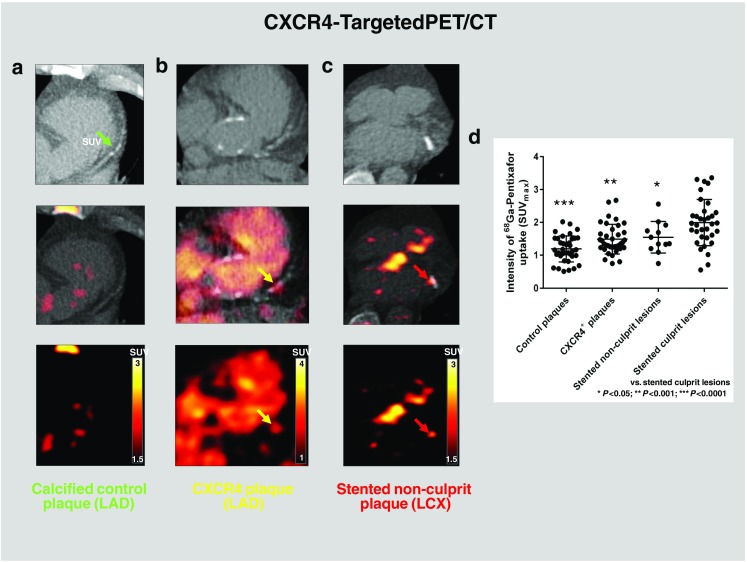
[^68^Ga]Pentixafor PET/CT identifies CXCR4 upregulation in various types of coronary lesions. a Calcified LAD plaque without visually identified uptake (control). b CXCR4^+^ LAD plaque, partially calcified on CT and with CXCR4 signal. c Focal CXCR4 upregulation at the site of a stented LCX nonculprit lesion fusing to the stent location. d Signal intensity of CXCR4 expression is highest in stented culprit lesions and lowest in calcified plaques without visually identified uptake (controls) (ANOVA, *P* < 0.0001). The images were obtained from different patients. Background subtraction was performed using individually adjusted thresholds for clearer visualization of tracer uptake
